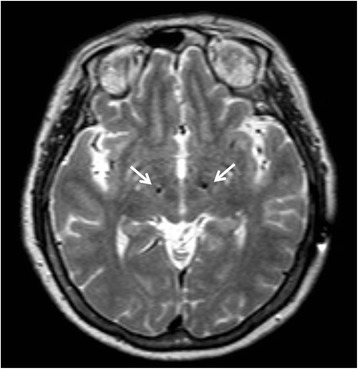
Post-surgery Brain MRI of the index patient. Arrows indicate the implanted locations of leads of DBS
